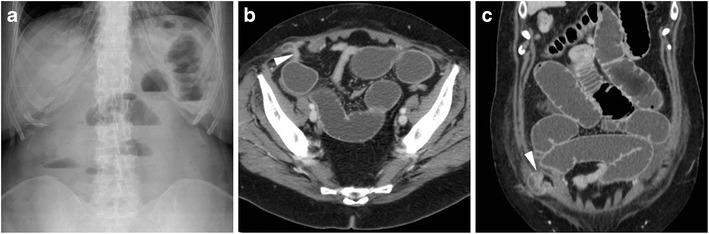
Small-bowel obstruction developing after recent LH. The patient was readmitted with plain radiographic (A) evidence of distended bowel loops with multiple fluid-fluid levels. CT (B, C) confirmed distended jejunal and ileal loops with abundant intraluminal fluid, caused by herniation (arrowhead) into a laparoscopic trocar access site
